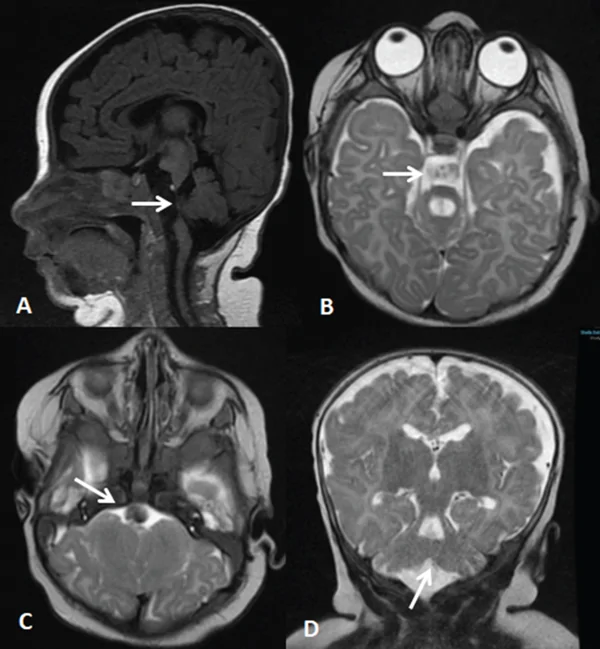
Magnetic resonance imaging (MRI) of the brain. (A) Sagittal T1‐weighted image demonstrates the absence of the pons, with a thin cord connecting the medulla oblongata, midbrain, and cerebellum (solid arrow). (B,C) Axial T2‐weighted images demonstrate the absence of the normal flow void of the basilar artery (solid arrows), suggesting possible aplasia or hypoplasia, and (D) coronal T2‐weighted image reveals hypoplastic cerebellar vermis and hemispheres (solid arrows), along with a prominent cisterna magna and distortion of the fourth ventricle.
